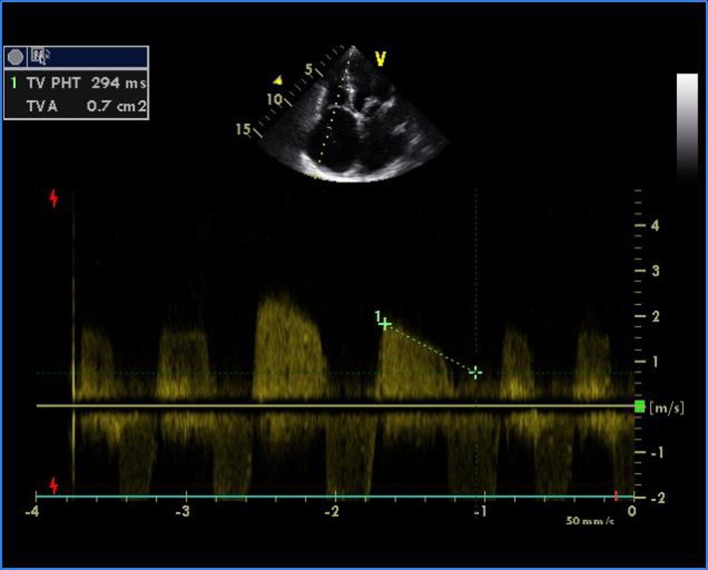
Transthoracic echocardiogram of apical four-chamber view showing severe tricuspid stenosis with valve areas of 0.7 cm^2^.
